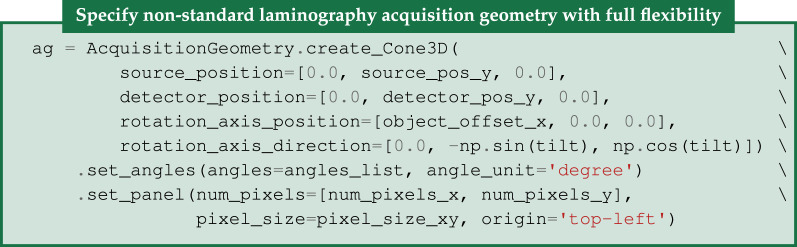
The data consist of 2512 projections of 798-by-574 pixels sized 0.508 mm in a 360° cone-beam geometry. We load the data with NikonDataReader and preprocess with a couple of Processor instances to prepare it for reconstruction. For reconstruction we use the GPU-accelerated cone-beam ProjectionOperator from ccpi.plugin.tigre and FISTA to solve equation (3.8) for the unregularized least-squares problem (LS) and non-negativity constrained TV-regularized least-squares (TVNN). For TVNN, we use FBP_TV from cil.plugins.ccpi_regularisation which implements a GPU-accelerated version of *g*_TV_, which is faster than, but otherwise equivalent to, using the native CIL TotalVariation. The full three-dimensional volume is reconstructed for LS and TVNN, and figure 10 shows a horizontal and vertical slice through both.
